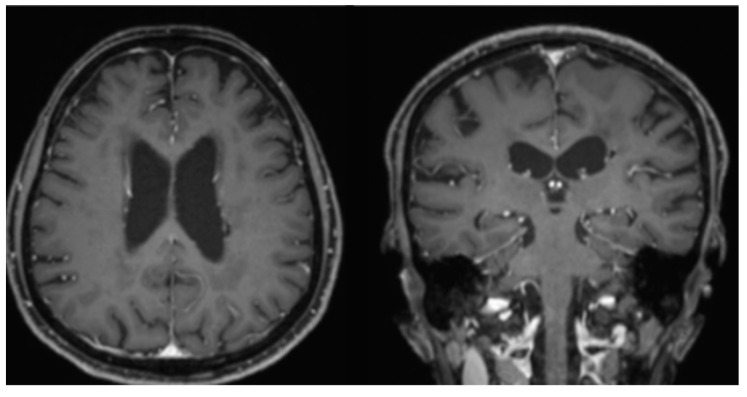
Brain MRI after 6 cycles of ibrutinib, showing resolution of small, enhanced lesion on post-contrast T1 images.
